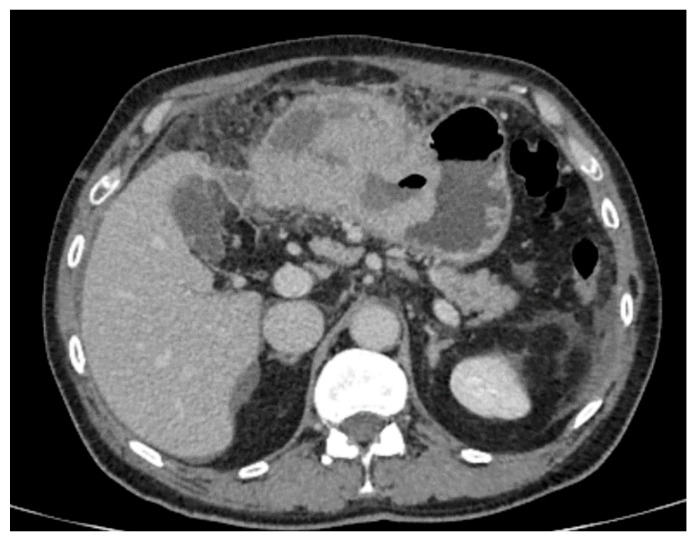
CT scan demonstrates massive necrotic tumor with ill-defined margins and disrupted mucosa with exulceration (HR GIST).
